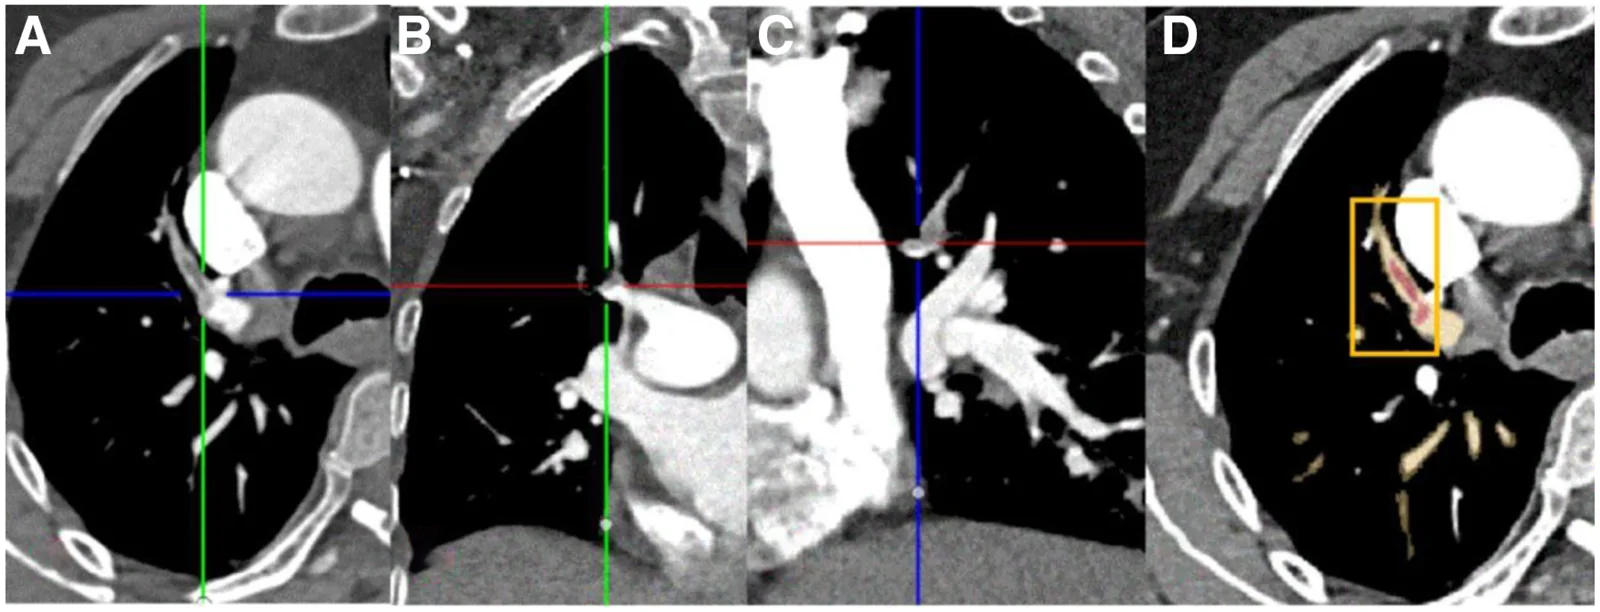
True-positive AI detection. Panels (A–C) show the thrombus on axial, coronal, and sagittal CTPA images, respectively; panel (D) shows accurate detection by the AI system. AI = artificial intelligence, CTPA, computed tomography pulmonary angiography.
